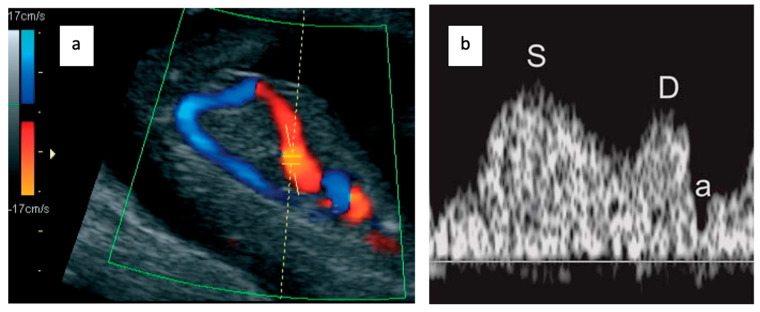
The ductus venosus color Doppler (a) and ductus venosus waveforms (b) showing the three phases: (S) systole, (D) diastole, and (a) atrial contraction.
